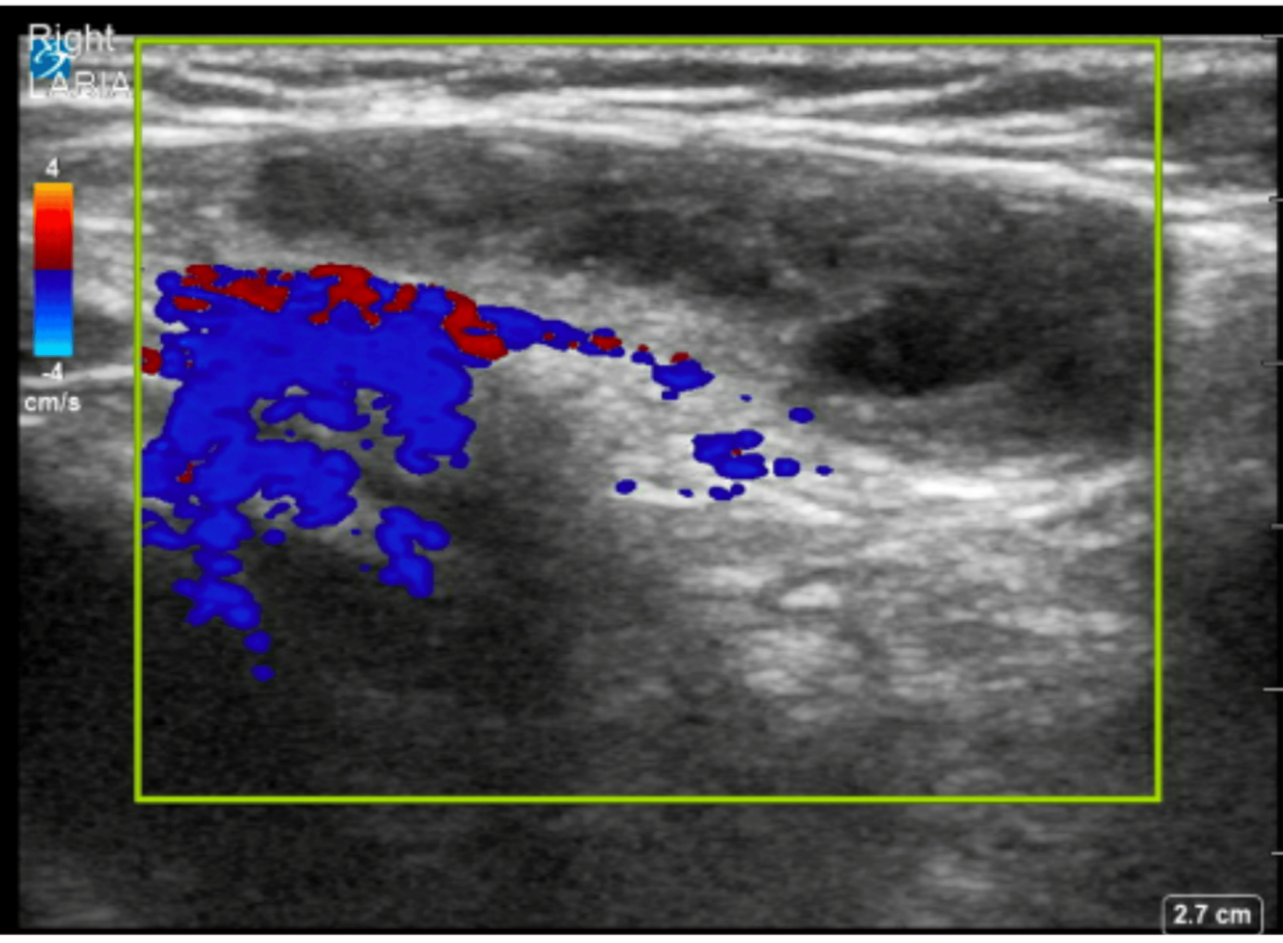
Point-of-care ultrasound of the right groin with color Doppler
